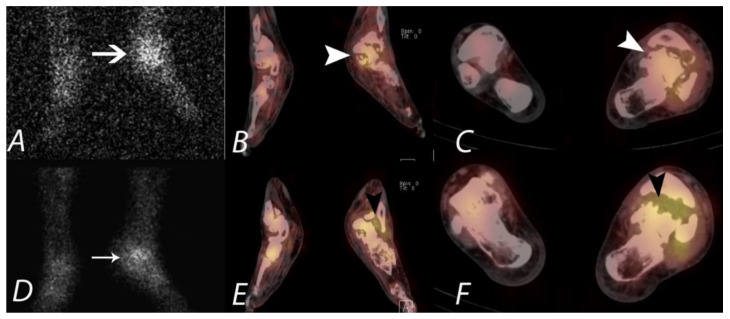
A seventy-year-old diabetic male patient with chronic left midfoot Charcot joint changes was evaluated for possible superimposed infection. Planar image (A), coronal fused axial image (B), and axial fused axial SPECT/CT image (C) from In-111 WBC scan demonstrate focal uptake localizing to mid and hind foot (large white arrow and white arrowheads), which is congruent with uptake identified on Tc-99m sulfur colloid images (D–F), supporting the presence of chronic inflammation and granulation tissue and not active infection (small arrow and black arrowheads).
